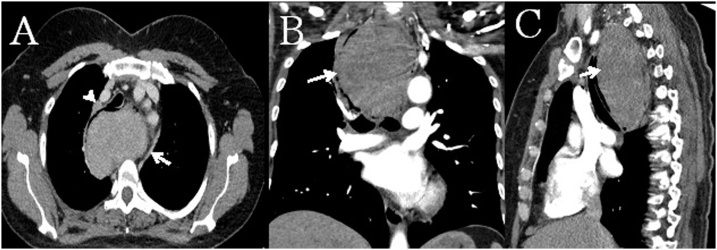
Computed Tomography (CT) Angiography of the chest with arterial and venous phase revealing a well-defined soft tissue mass (*arrow*) within the lumen of the esophagus at its proximal third measuring around 7.8 × 5.4 x 10.5 cm in its maximal transverse, AP, and craniocaudal dimensions respectively. It shows a smooth contour with mild enhancement on the arterial phase, which increases on the venous phase images. There is no extraluminal extension. A 7 mm lymph node (*arrowhead*), adjacent to the esophagus in the upper mediastinum, is also noted. (A: axial; B: coronal; C: sagittal cuts).
